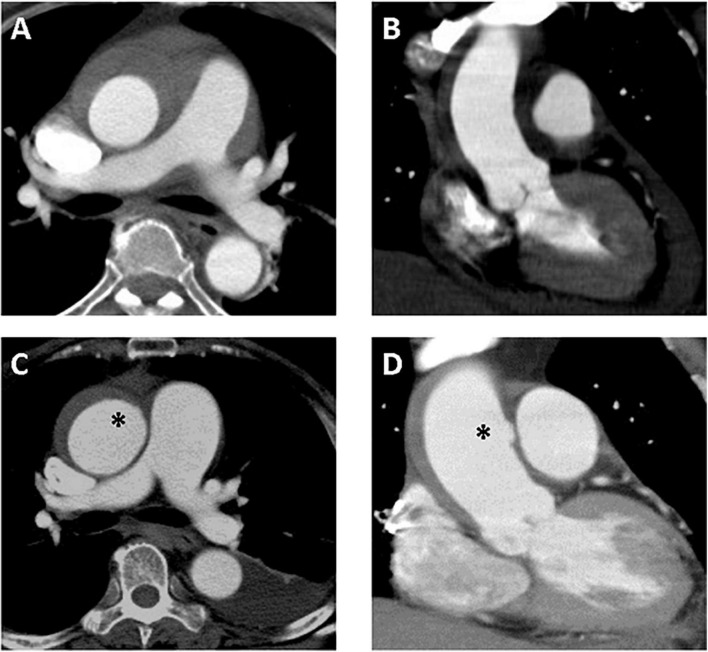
A 61-year-old woman with TAIMH and newly developed ULP. Type A intramural hematoma (thickness 12 mm) was identified on initial contrast-enhanced CT scan and no focal intimal disruption was visible in the axial (A) and coronal view (B). Follow-up CT scan obtained on sixth day of admission showed an irregular margin of aortic wall (asterisk) in the axial view (C) that a newly developed ULP (asterisk) was confirmed in the coronal view (D). The patient suffered from sudden death on the 12th day of admission. CT, computerized tomography; TAIMH, type A aortic intramural hematoma; ULP, ulcer-like projection.
